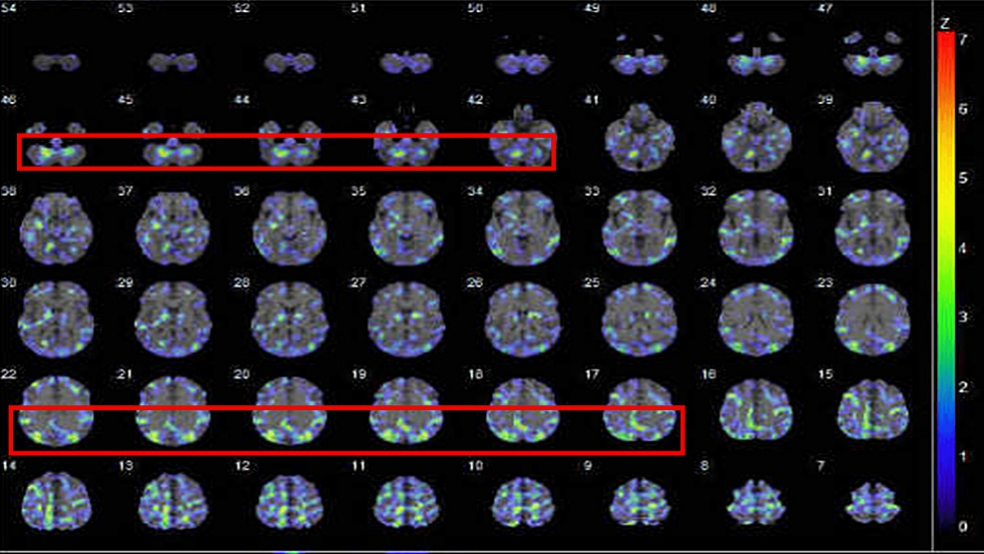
Brain N-isopropyl-p-(123I)-iodoamphetamine (IMP) single-photon emission computed tomography (SPECT) findings. The 123I-IMP-SPECT examination performed three months after the onset of the patient's SARS-CoV-2 infection indicated hypoperfusion in the bilateral cerebellar dentate nuclei and occipital lobes. The areas of hypoperfusion are shown on three-dimensional surface projection (3D-SSP: decrease).
